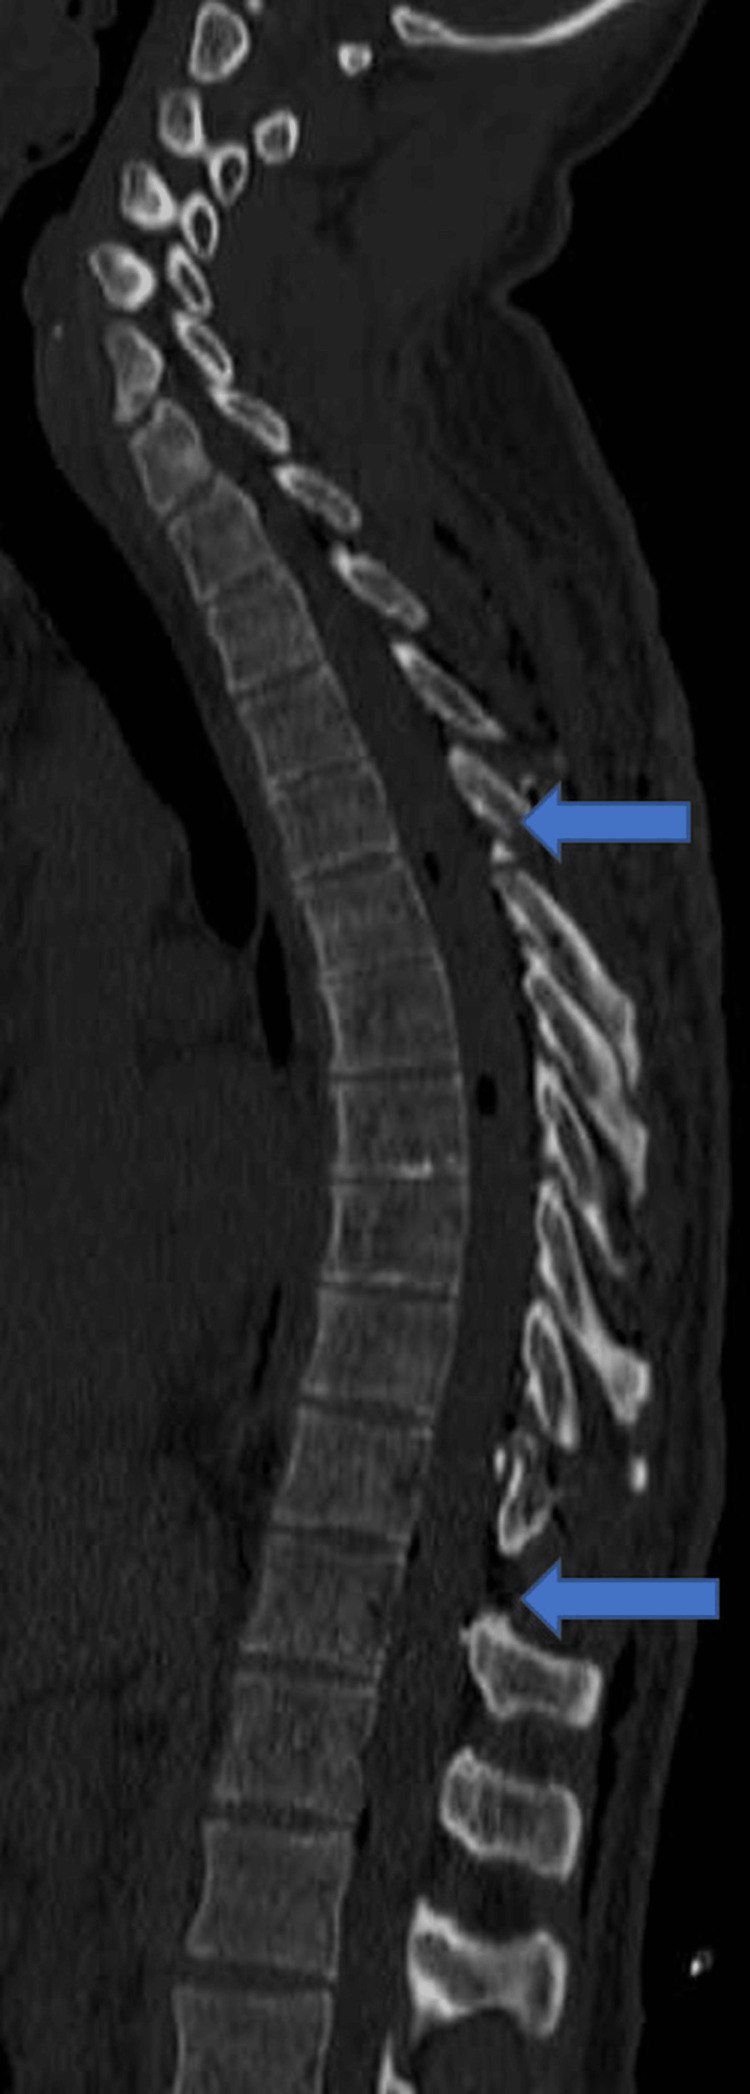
Postoperative CT scan of the thoracic spine showing the borders of laminoplasty Postoperative sagittal CT scan of the thoracic spine showing that laminoplasty was performed. The blue arrows indicate the borders of laminoplasty.
